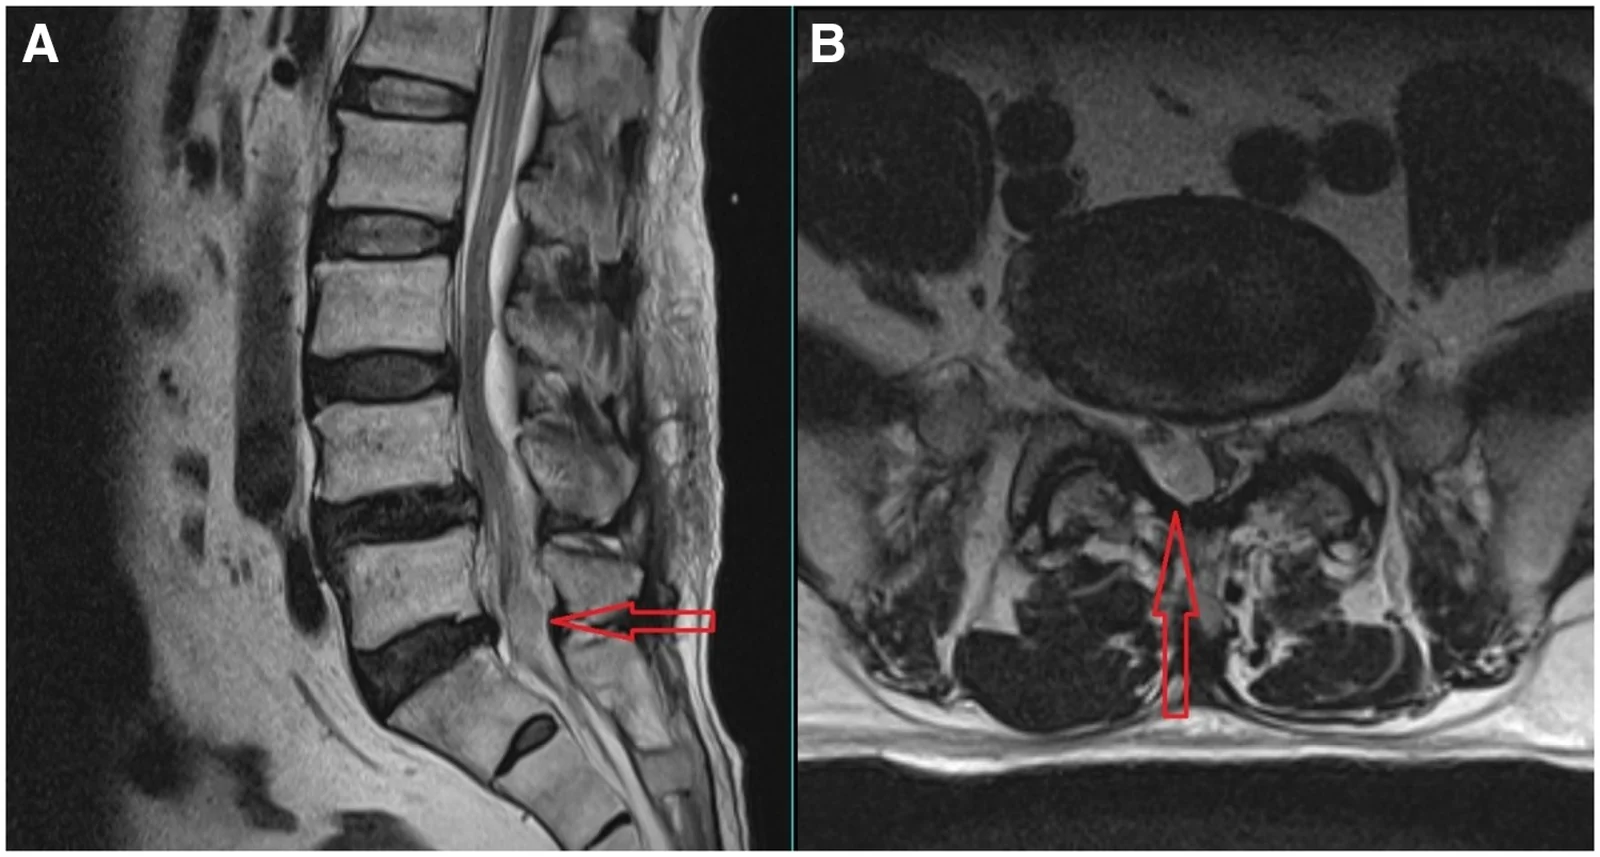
Postoperative lumbar spinal MRI showing a distal epidural hematoma. (A) T2-weighted sagittal image demonstrating a spinal epidural hematoma at the L5–S1 level, distal to the operative site, compressing the thecal sac (arrow); (B) corresponding T2-weighted axial image showing the epidural hematoma at the same level (arrow). MRI = magnetic resonance imaging.
